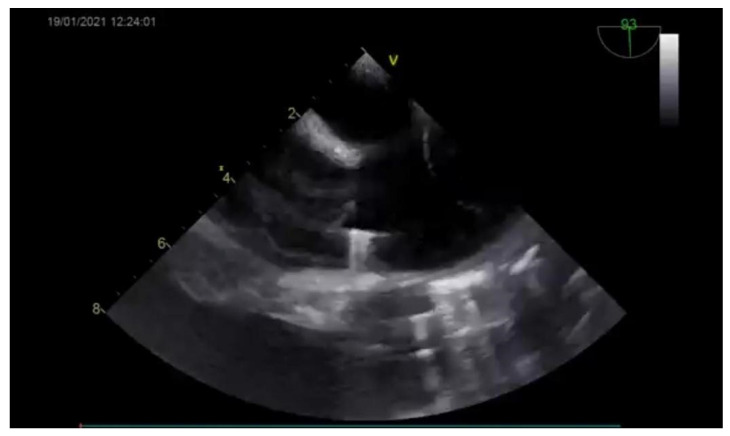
Preoperative echocardiography (bicaval view) showing the ASD.
